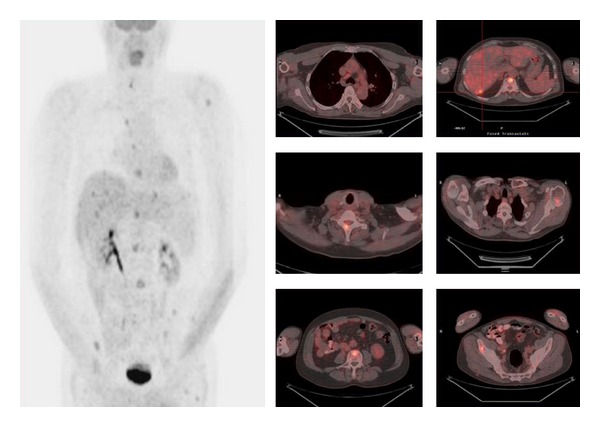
[^18^F]FDG-PET and [^18^F]FDG-PET/CT images in a 59-year-old man with known MTC, as part of MEN IIA syndrome and calcitonin levels of 4800 pg/mL, about 2 years after the initial treatment. Images show increased uptake of ^18^FDG in multiple hepatic lesions (SUVmax: 6), a precarinal lymph node (SUVmax: 2.9) and in multiple bone lesions (SUVmax: 6.1).
